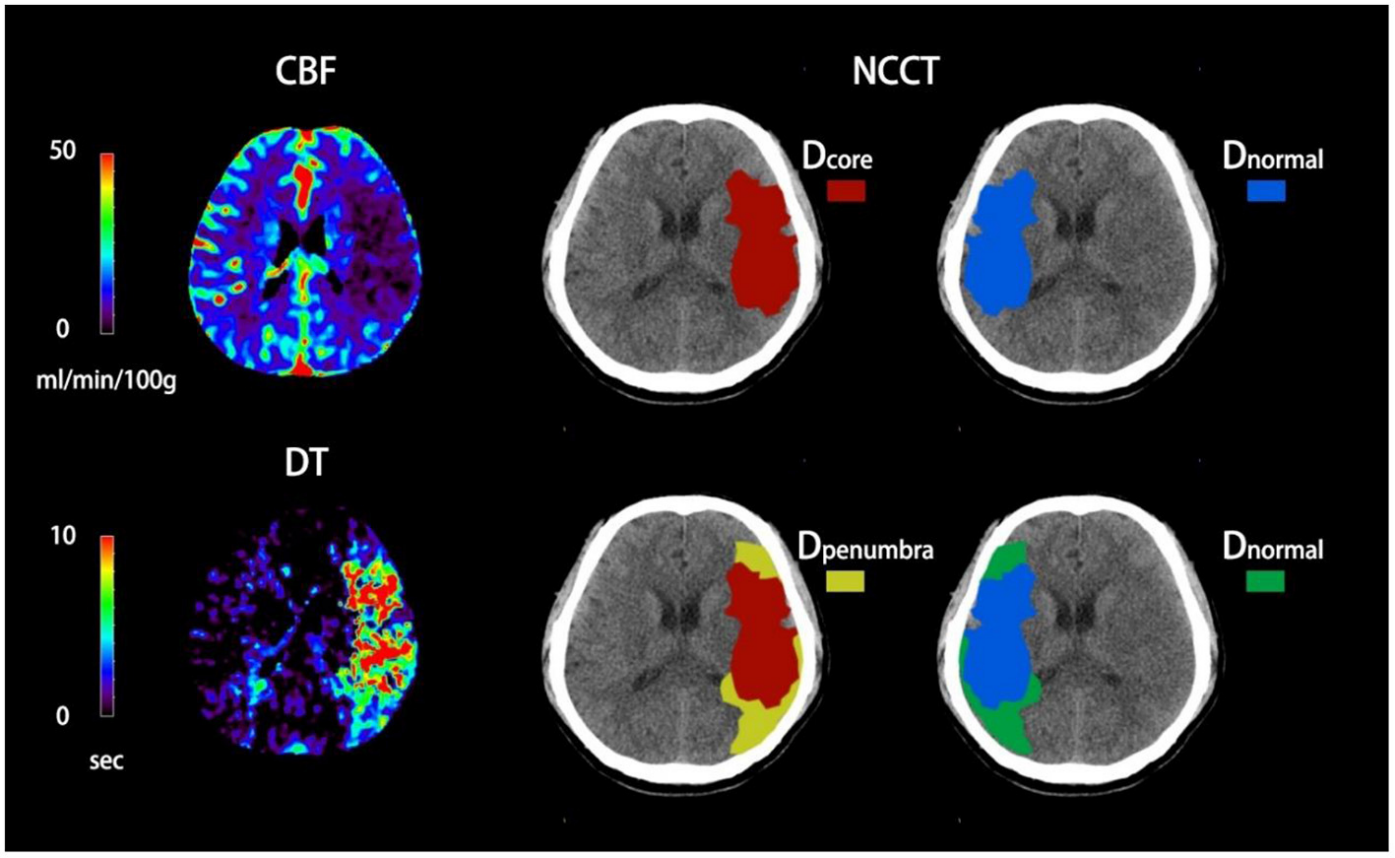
Quantification of NWU-core and NWU-penumbra in admission non-contrast computed tomography (NCCT). The ischemic core and penumbra were identified by cerebral blood flow (CBF) and delay time (DT) maps based on computed tomography perfusion (CTP) with the setting of different thresholds. The mean density of the ischemic core, ischemic penumbra, and normal tissue derived from the mirrored contralateral were then calculated.
